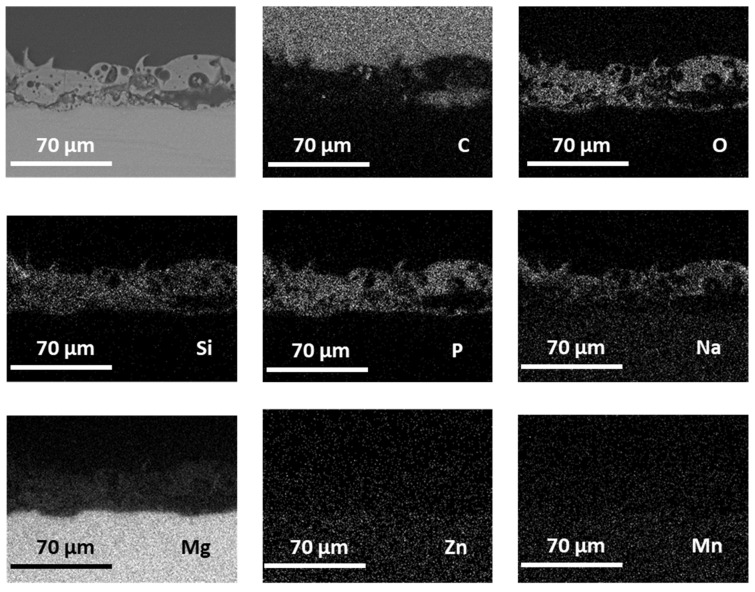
BSE image (top left) and X-ray EDS elemental maps of the PEO-treated ZM21 extruded profile (polished cross section; the presence of C is due to the mounting resin).
